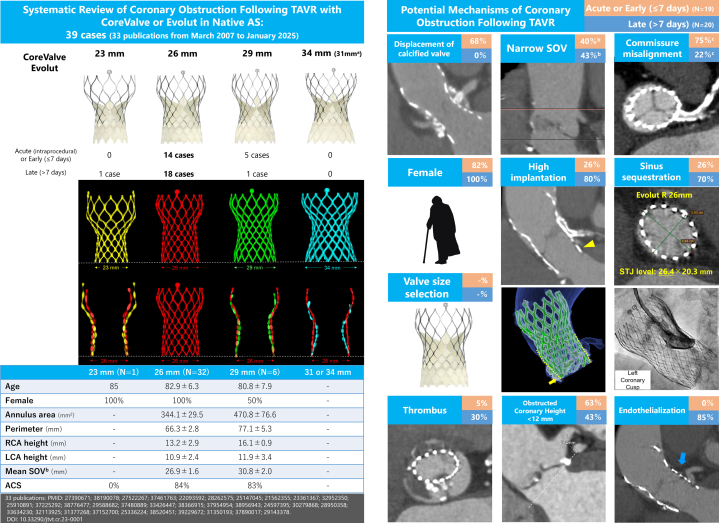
CoreValve/Evolut Sizes and Factors Associated with Coronary Obstruction Following Transcatheter Aortic Valve Replacement in Native Aortic Stenosis ^a^Maximum CoreValve size: 31 mm; Evolut up to 34 mm. ^b^Narrow SOV defined as <25 mm (23-mm valve), <27 mm (26-mm valve), and <29 mm (29-mm valve) per Medtronic IFU; SOV data available in 24/39 cases. ^c^Commissure alignment reported in 13/39 cases. All valve sizes were superimposed on the 26-mm valve (red), with bases aligned. The 26-mm valve showed the most cylindrical lower shape. High implantation, defined as <3 mm depth from the left coronary cusp (yellow arrows), allowed further expansion above the outer skirt (yellow dot lines). Aortography and CT revealed no left coronary flow due to sinus sequestration with endothelialization (blue arrow). The 26-mm Evolut R expanded elliptically (26.4 × 20.3 mm at STJ), contacting the aortic wall directly with minimal residual space between the valve and surrounding structures. ACS = acute coronary syndrome; AS = aortic stenosis; LCA = left coronary artery; RCA = right coronary artery; SOV = sinus of Valsalva; STJ = sinotubular junction; TAVR = transcatheter aortic valve replacement.
